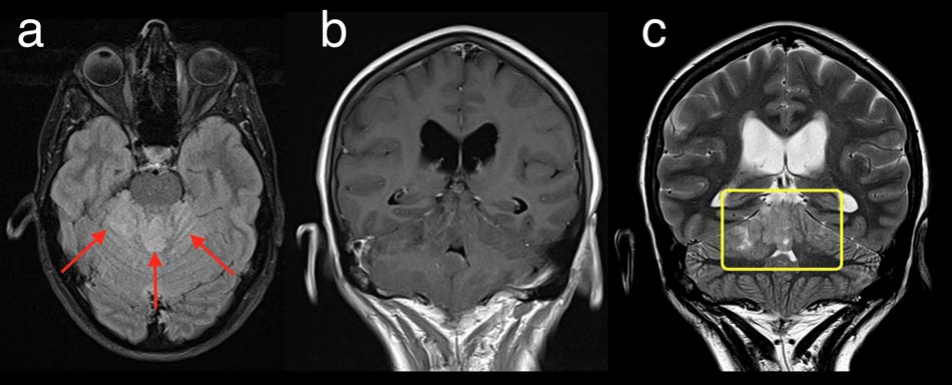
Axial T2 FLAIR (a), Coronal T1 post-contrast (b) and T2 (c) demonstrates a T2 FLAIR hyperintense mass lesion centred on the vermis (red arrows) in keeping with medulloblastoma. No enhancing component was identified (b) with heterogenous T2 signal within the vermis (yellow box).
